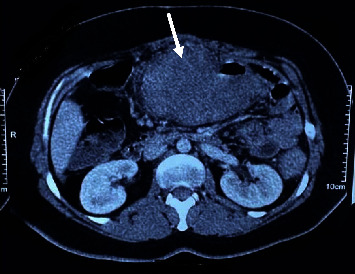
Abdominal CT scan with contrast shows a huge mass in the transverse colon.
